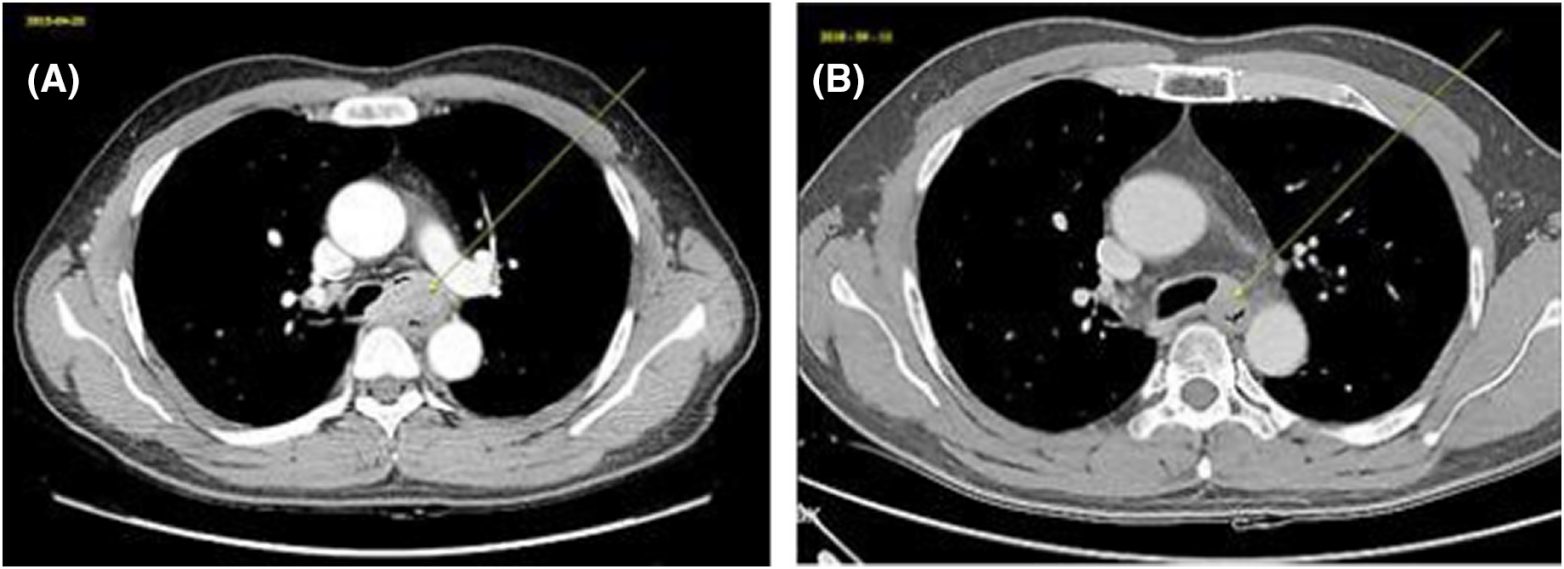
Chest computed tomography (CT) scan. (A) Pretreatment Chest CT scan showing tumor located at the carina level in the distal trachea with total obstruction to the left main bronchus and partially pushed right main bronchial lumen (arrow)—axial view. (B) After 3 years of completion of CCRT, Chest CT scan showed initial large polypoid mass was significantly decreased in size.
